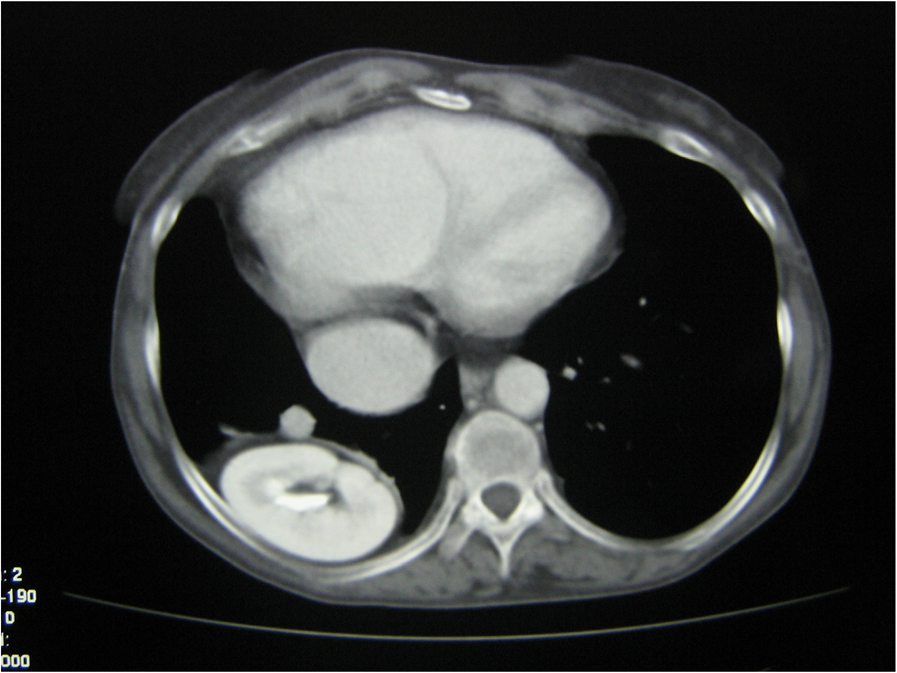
Computed tomography scan. Part of the right kidney and an enlarged inferior vena cava are shown, flowing into the also enlarged right atrium.
